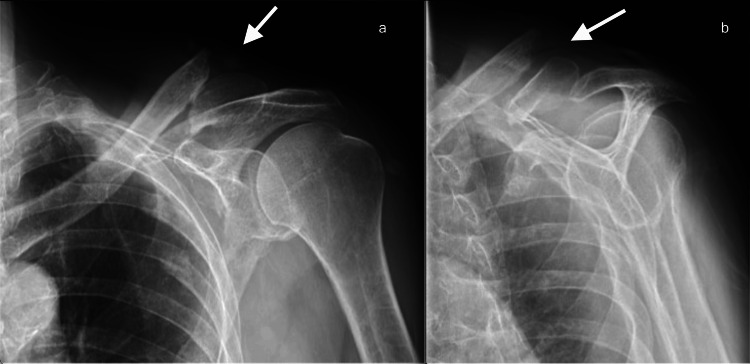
Radiographs of left shoulder: (a) anteroposterior (AP) and (b) scapular Y views showing distal clavicle non-union with superior displacement of the medial fragment
